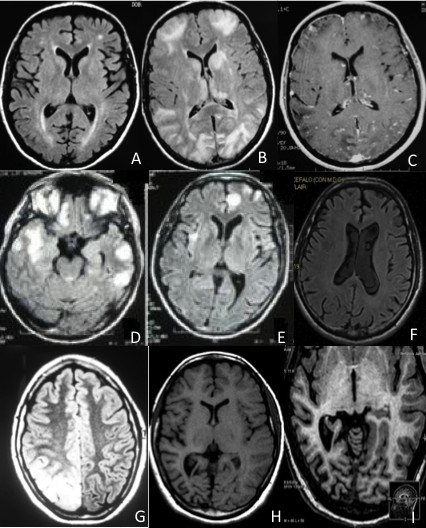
Brain MRI in our series. A (patient 1, at entry): hyperintense spots in axial FLAIR sequence involving periventicular and subcortical white matter. B, C (patient 1, one month later): axial FLAIR sequence showing multiple cortico-subcortical hyperintense lesions, some of which enhanced after gadolinium administration (axial T1: C). No meningeal enhancement is observed. D, E (patient 2, at entry): multiple nodular cortico-subcortical FLAIR hyperintense lesions involving fronto-temporal and insular regions. F (patient 2, three years later): axial FLAIR sequence revealing no abnormality. G (patient 3, three years after disease onset): axial FLAIR sequence showing a vast hyperintense cortico-subcortical temporo-occipital lesion. H, I (patient 3, two years after treatment onset with azathioprine): axial T1 revealing mild cortico-subcortical atrophy involving right temporal and occipital lobes with dilatation of posterior horn of right lateral ventricle. Magnification of right posterior atrophy by axial IR (I).
